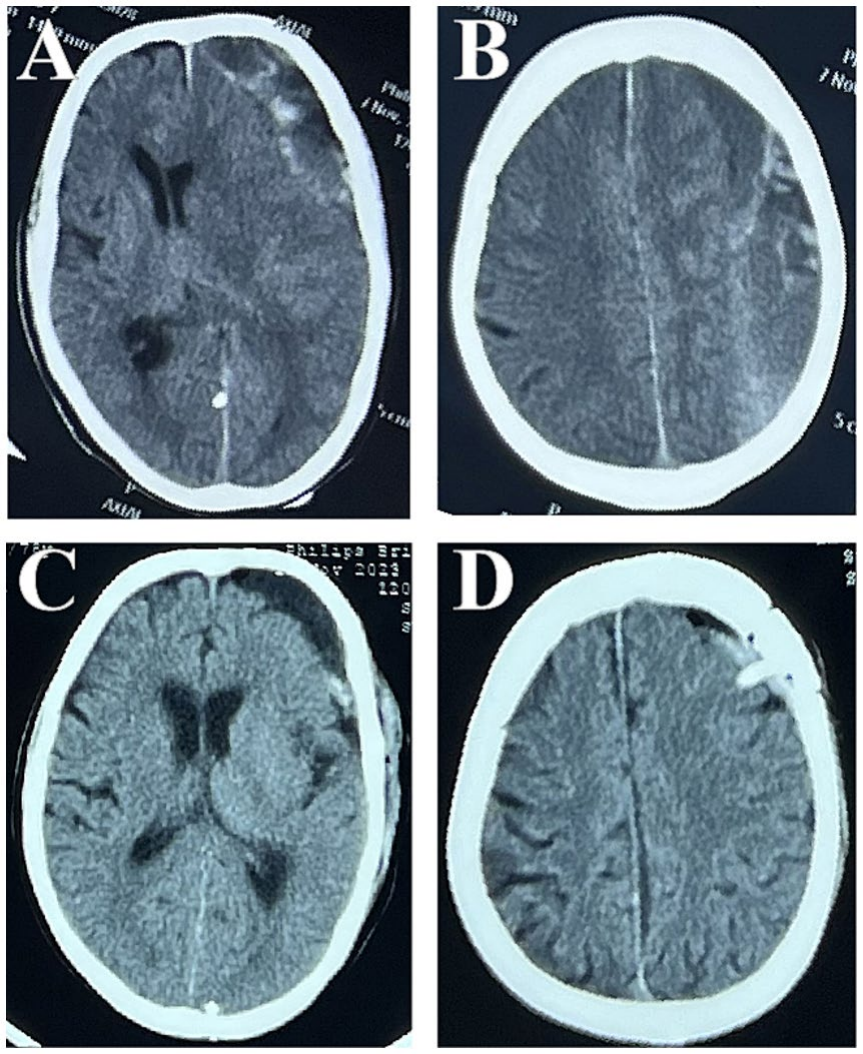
Preoperative CT examination showed a large left-sided front temporal occipitoparietal sCSDH with prominent midline shift; the subdural cavity contained several fibrous septa (A,B). Postoperative CT shows the evacuated haematoma with expected fluid (C,D).
